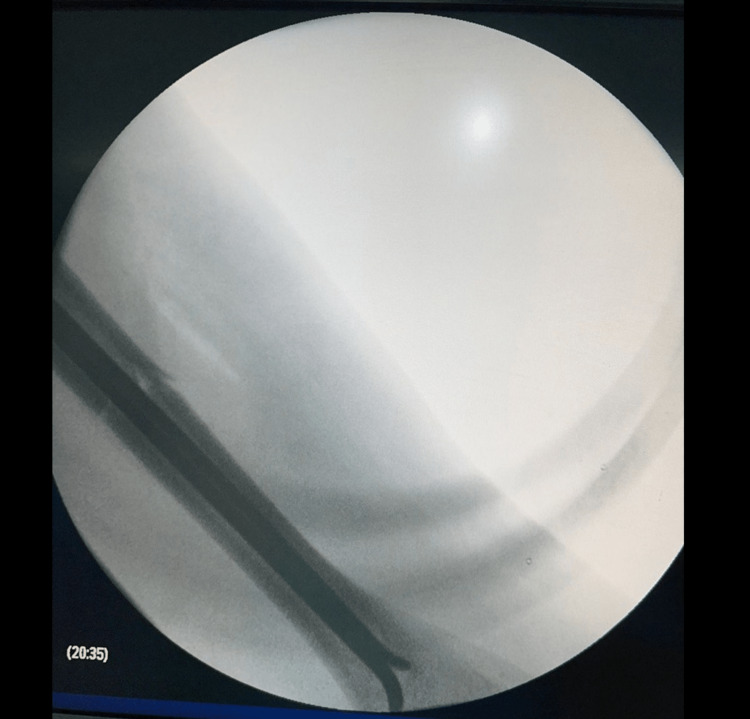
Intraoperative X-ray with intramedullary nails
